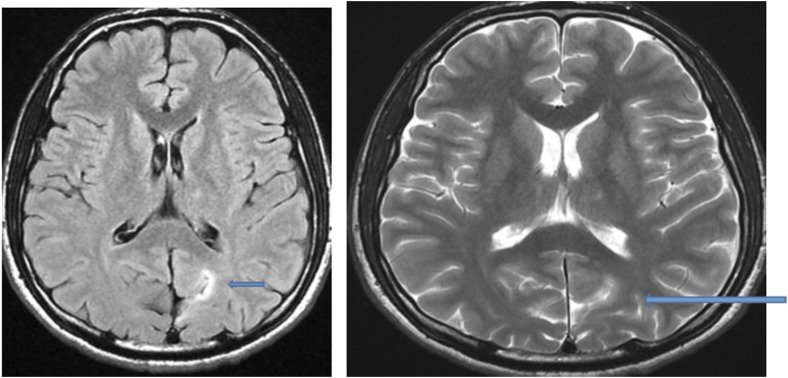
MRI of the brain of a 13-year-old male child: Axial FLAIR and T2-weighted image showing hyperintense focal thickening at the left occipitoparietal sulcus (arrow) compatible with focal cortical dysplasia.
